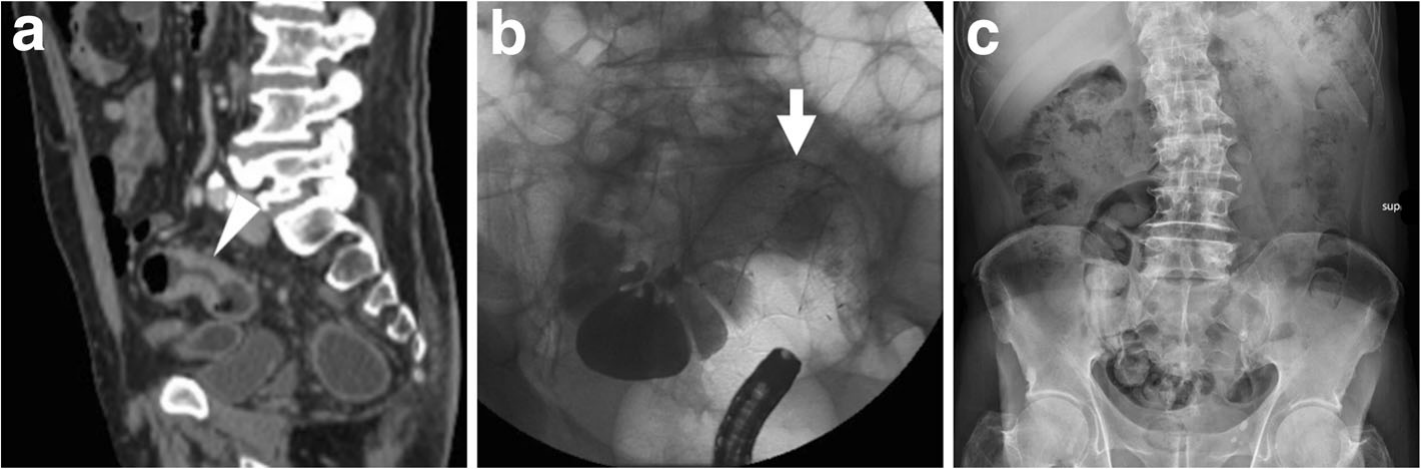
Spontaneous stent migration. In a patient with endoscopically impassable sigmoid CRC (arrowhead in initial water enema CT, a) with liver metastases (not shown) at diagnosis, multidisciplinary consultation opted to postpone surgical resection after neoadjuvant chemotherapy. A SEMS (thick arrow) was positioned endoscopically under radiologic guidance (b) and allowed relief of LBO, clinical improvement and start of chemotherapy. A month later, the patient experienced evacuation of the SEMS with stools and radiographs (c) confirmed SEMS loss [adapted from Open Access Ref. [38]
